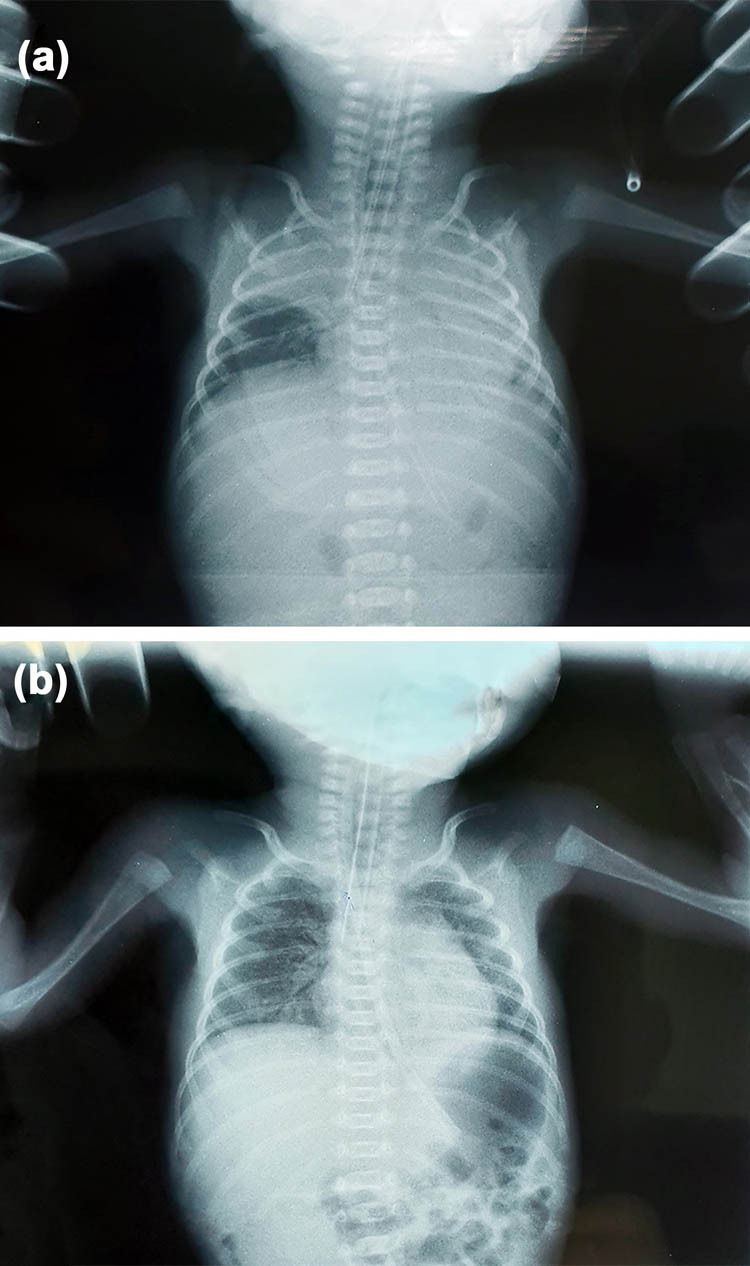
Chest X-ray of the newborns. (a) Image shows decreased transparency of the pulmonary parenchyma to the left with a clearly limited presence in the upper lobe area to the right in the first newborn; (b) chest X-ray shows individual paracardial and basal shadings of the pulmonary parenchyma in the second newborn.
